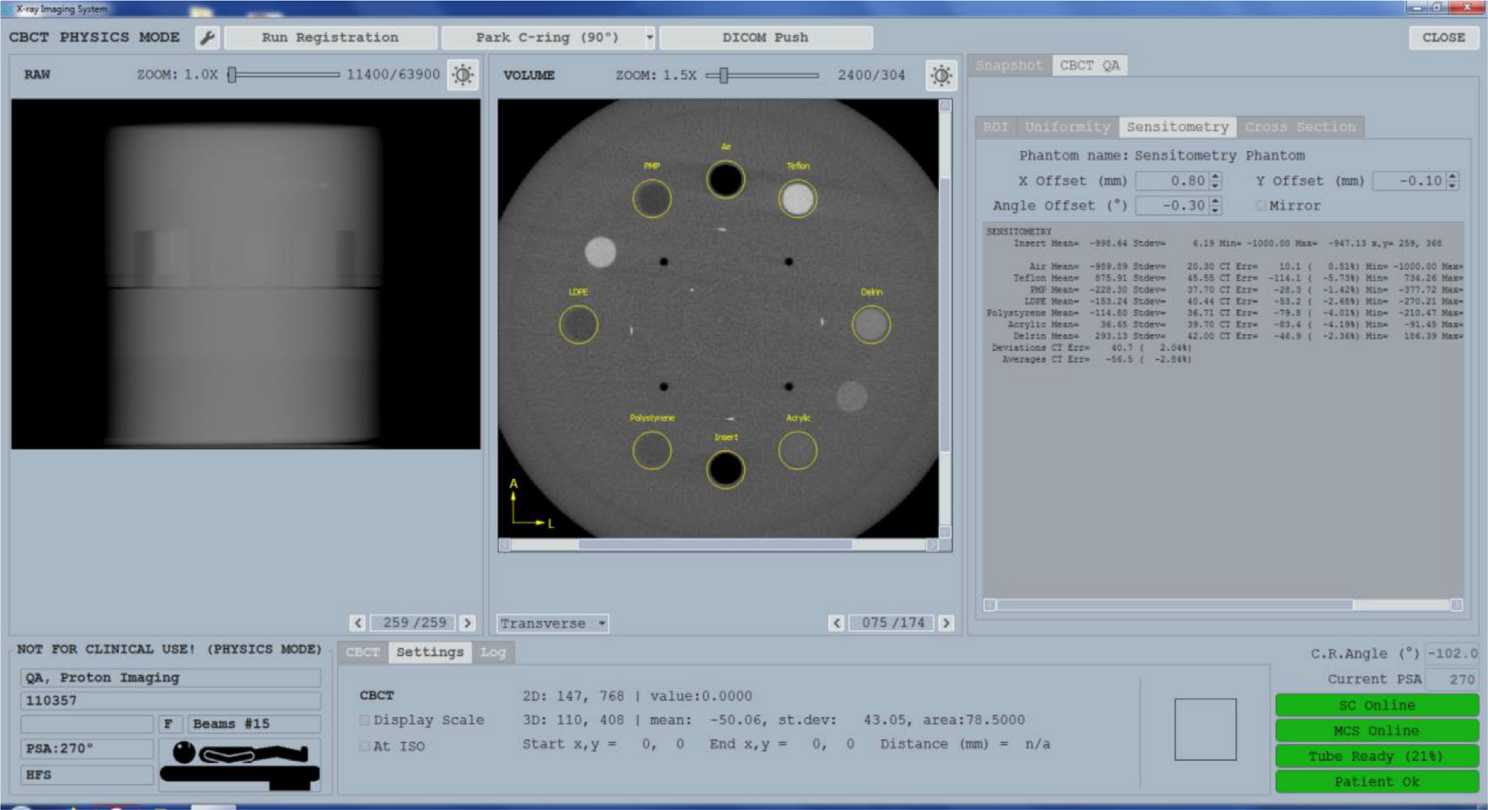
XIS CBCT physics QA mode for the sensitometry test. QA, quality assurance.
